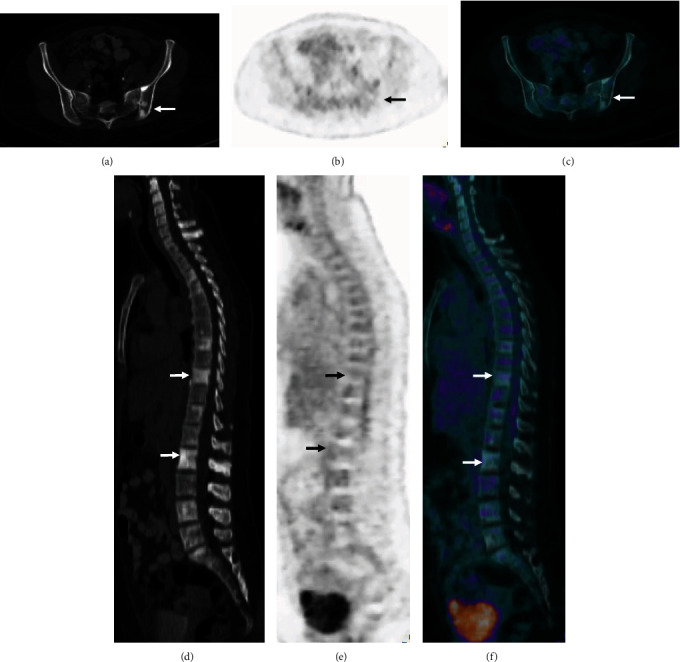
Axial low dose CT (a), FDG PET (b), and fused FDG PET/CT (c) images demonstrate sclerotic bone lesions in the bony pelvis with no significant FDG uptake (arrows). Sagittal CT (d), FDG PET (e), and fused FDG PET/CT (f) images demonstrate sclerotic bone lesions throughout the spine with no significant FDG uptake (arrows).
